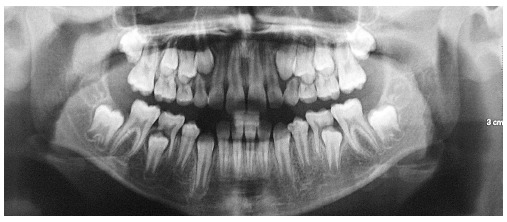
Panoramic radiograph at 11 years and one month old.
